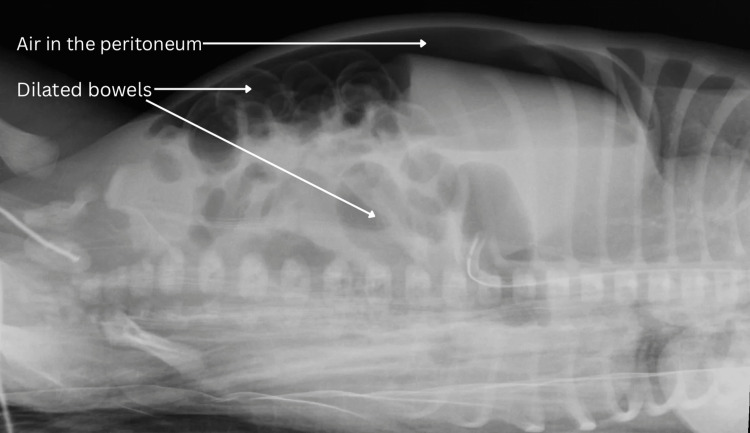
Repeated abdominal radiographs showing pneumoperitoneum Repeat radiographs demonstrated free intraperitoneal air, best seen on the lateral decubitus, lateral view, consistent with pneumoperitoneum. Multiple dilated bowel loops are also seen. These findings were concerning for bowel perforation and prompted urgent surgical intervention.
